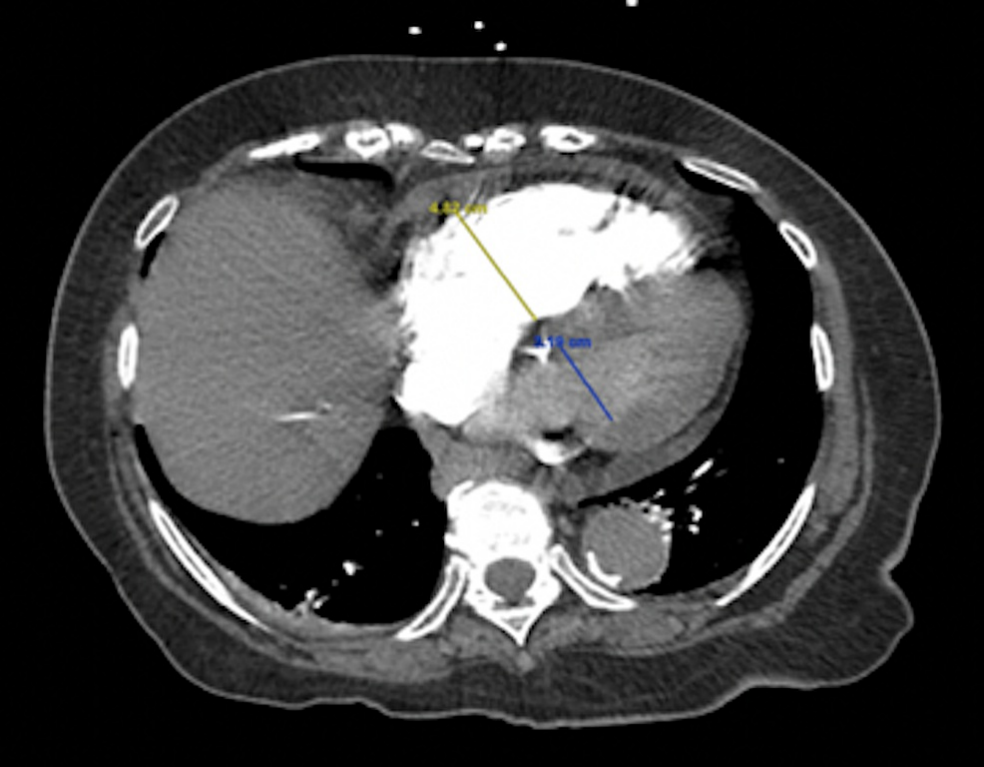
CT angiography of chest showing an increased right ventricular:left ventricular ratio of 1.5
